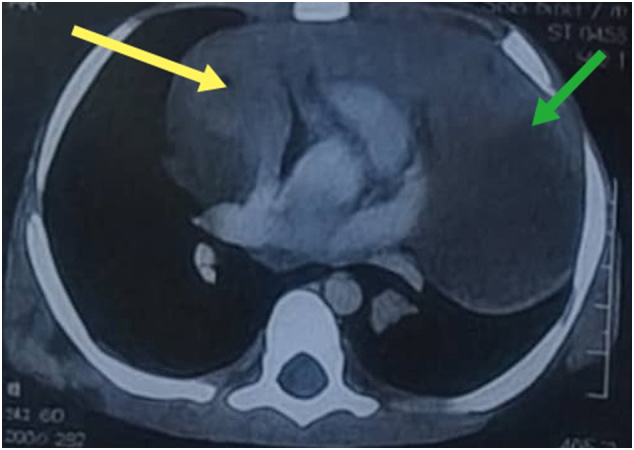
Axial chest computed tomography (CT) scan demonstrating a left-sided thoracic mass (green arrow) associated with mediastinal shift toward the right side (yellow arrow). (For interpretation of the references to colour in this figure legend, the reader is referred to the web version of this article.)
